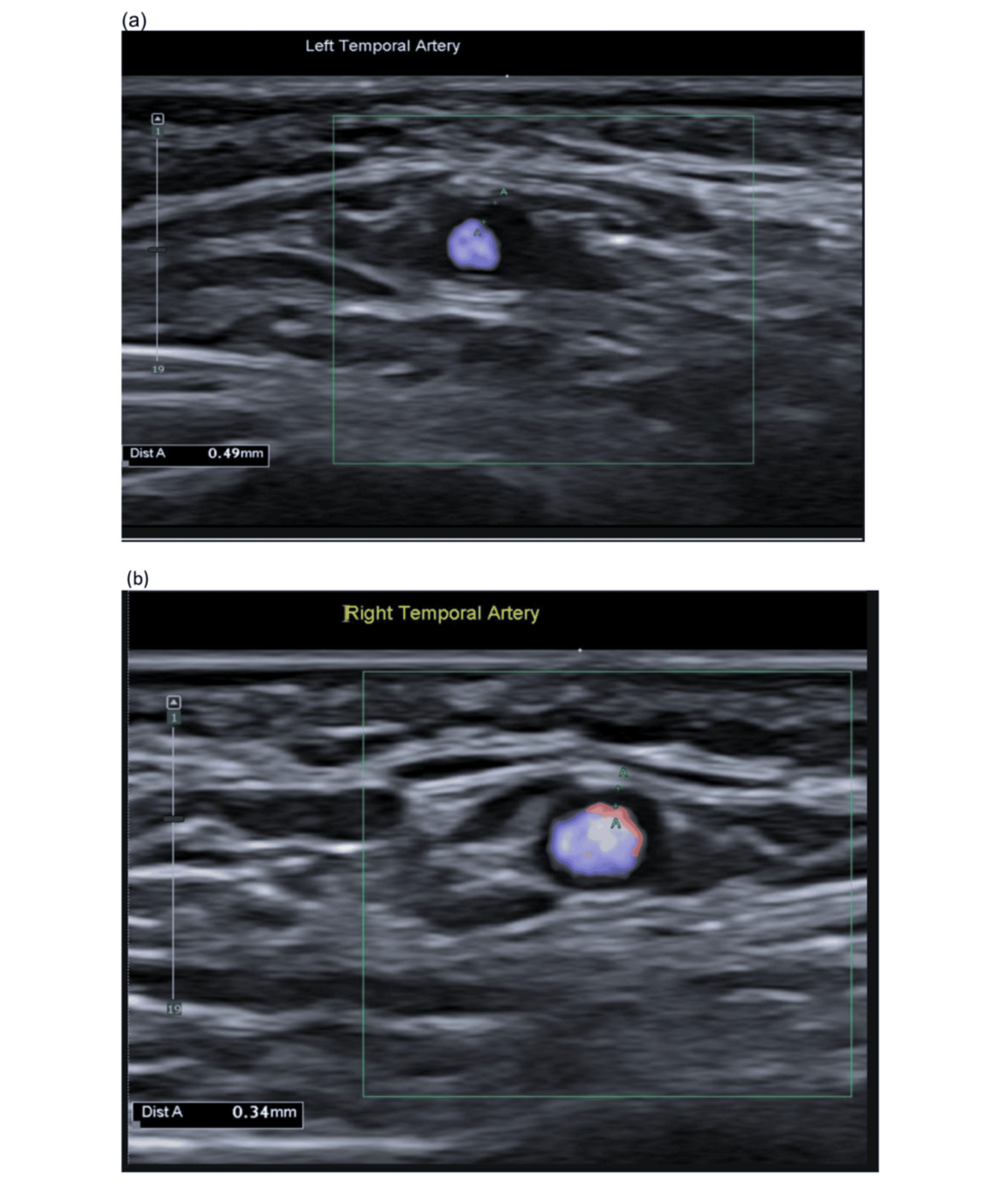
a-b: Temporal artery ultrasound showing halo signs in the left (0.49 mm) and right (0.34 mm) temporal arteries, including the involvement of the right parietal branch, consistent with inflammatory changes seen in giant cell arteritis.
